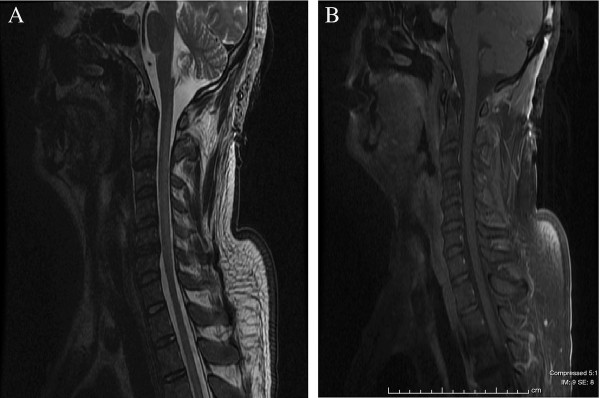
Magnetic resonance imaging of the cervical spine two months after the image in Figure1was taken. (A) T2 sequence. (B) T1 post-contrast image.
